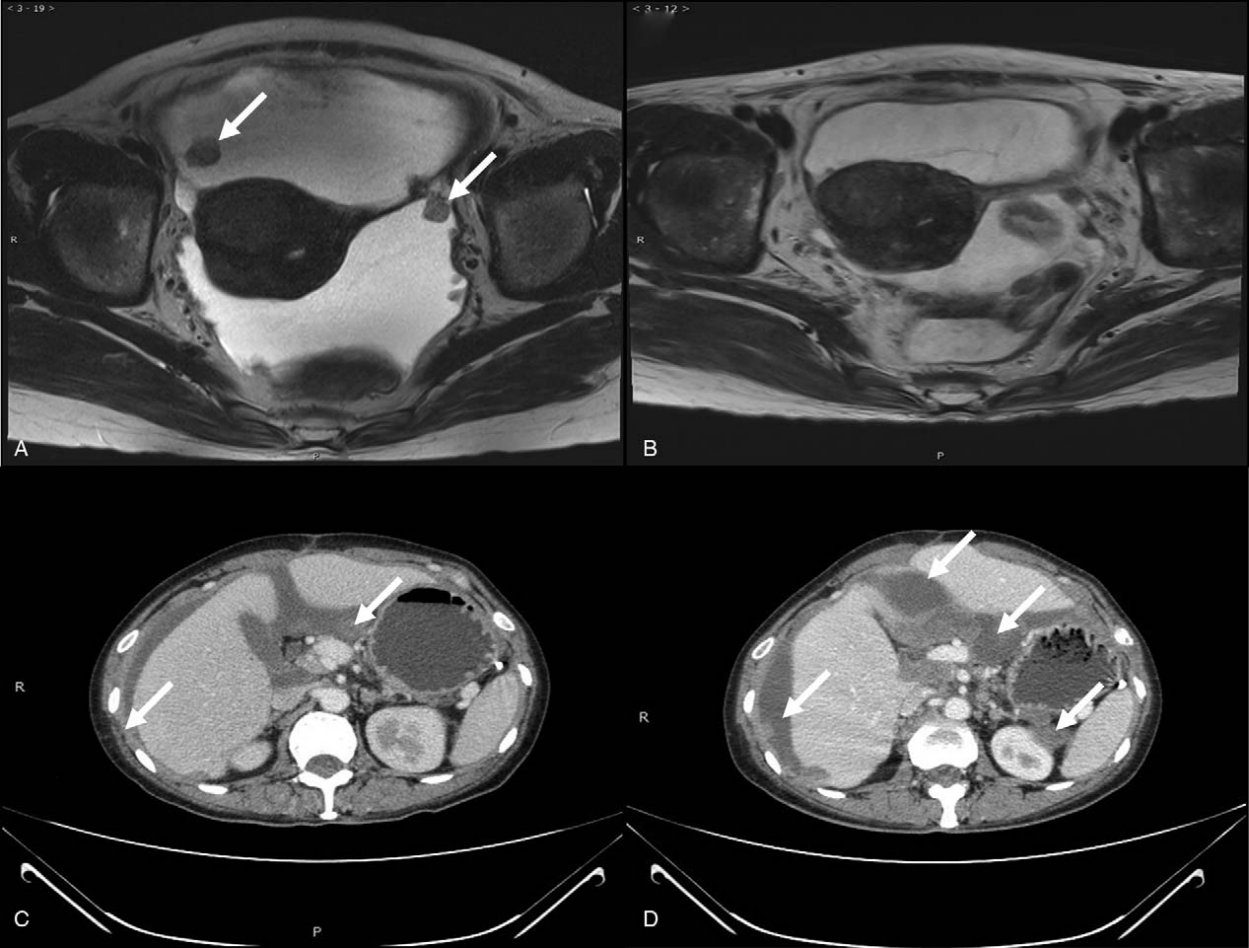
Results of pelvic computerized tomography (CT) and magnetic resonance imaging (MRI) of the patient. (A) Pelvic MRI before treatment which showed large amount of ascites and lesions (white arrows). (B) Pelvic MRI after 2 courses of chemotherapies (paclitaxel plus carboplatin) which demonstrated largely reduced ascites and lesions. (C) Abdominal CT and observed recurrent masses of soft-tissue density in pelvic and liver (white arrows) after the fifth chemotherapy (paclitaxel plus carboplatin). (D) Re-examination of abdominal CT found more and larger lesions after 8 courses of chemotherapies (5 courses of paclitaxel plus carboplatin and 3 courses of liposomal doxorubicin (white arrows).
